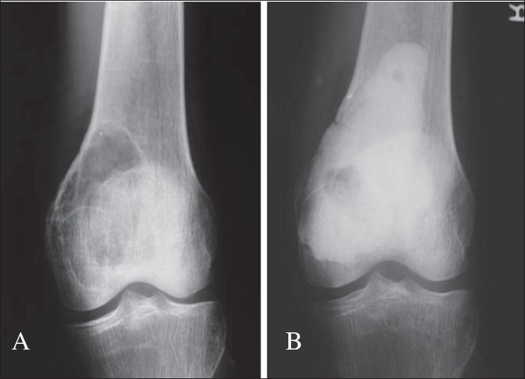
A) A.P. X-ray of a case of GCT lower end of femur, B) Treated with ‘extended intralesional curettage” and cementation
